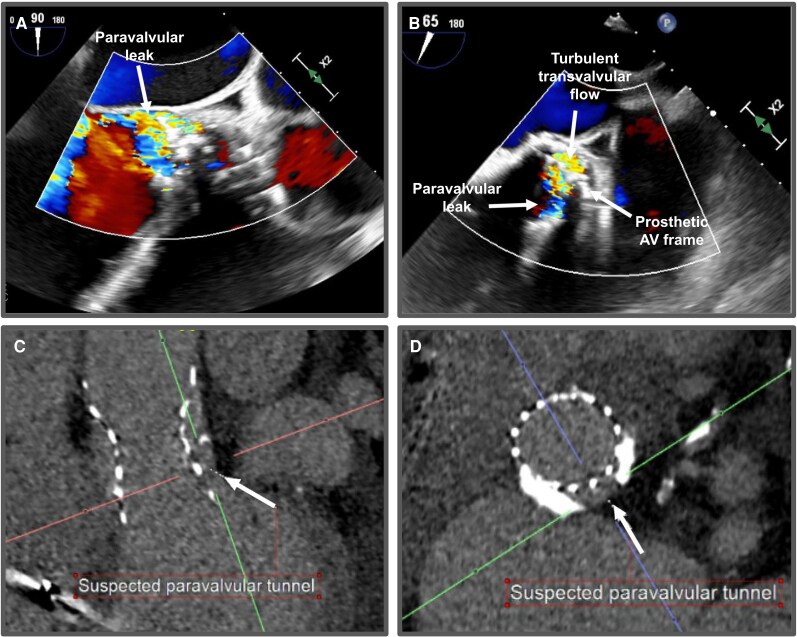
Multimodality imaging demonstrating concomitant paravalvular and transvalvular regurgitation: *(A and B)* TEE with colour Doppler, long- and short-axis views, demonstrating mixed-mechanism regurgitation, with severe paravalvular flow adjacent to the prosthetic frame, and severe transvalvular regurgitation centrally. *(C and D)* CTA, long- and short-axis reconstructions, demonstrating a self-expandable transcatheter aortic valve prosthesis with a discrete paravalvular regurgitant tunnel adjacent to the valve frame and located between two bulky calcific annular nodules.
